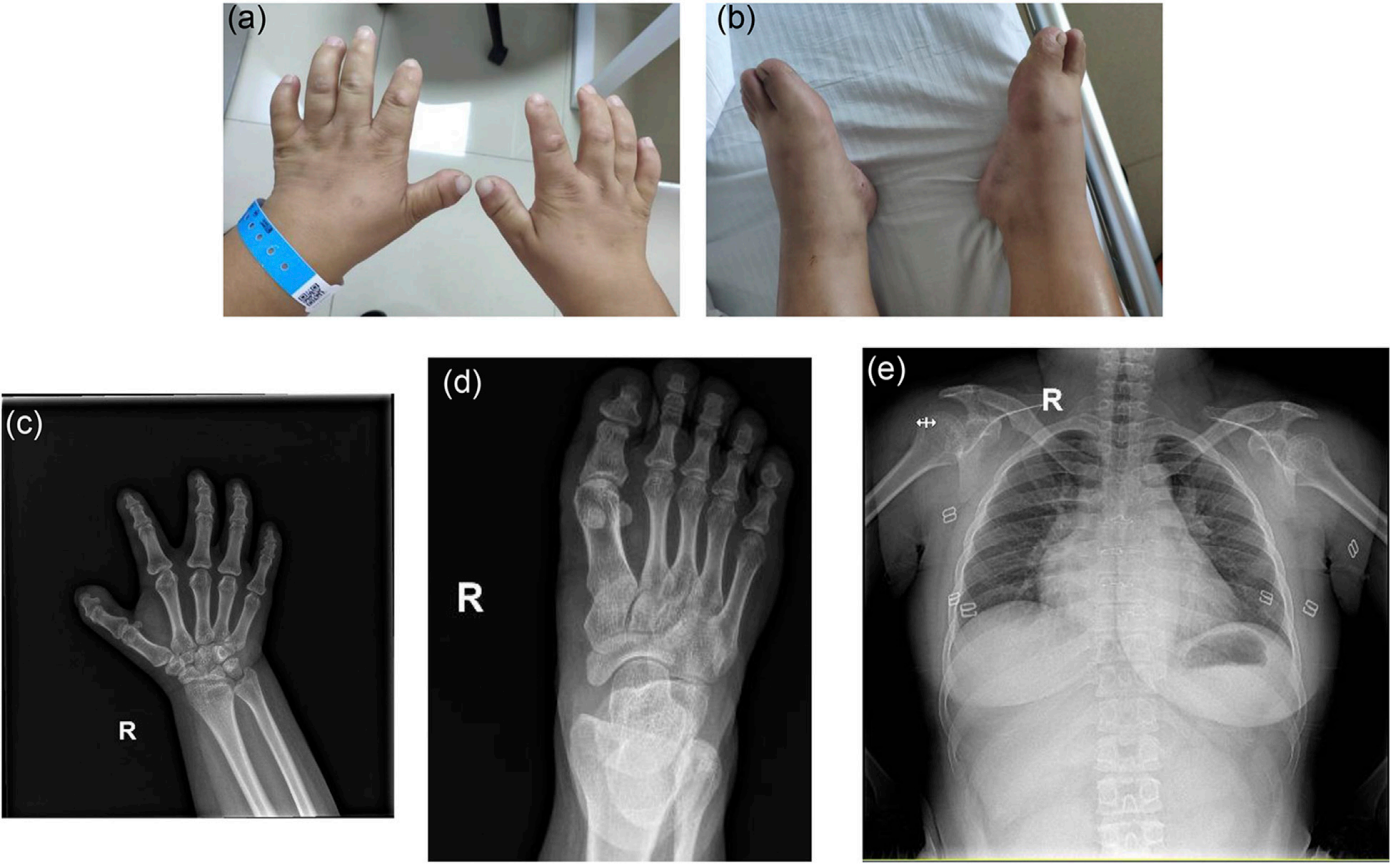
Clinical features of the patient on physic and radiographic examinations. (A,B) Note the short hands and feet. (C,D) Digital radiography reveals short tubular bones and cone shaped epiphyses in extremities (R, right). (E) Note the enlarged cardiac shadow and sternum closure metal wires.
